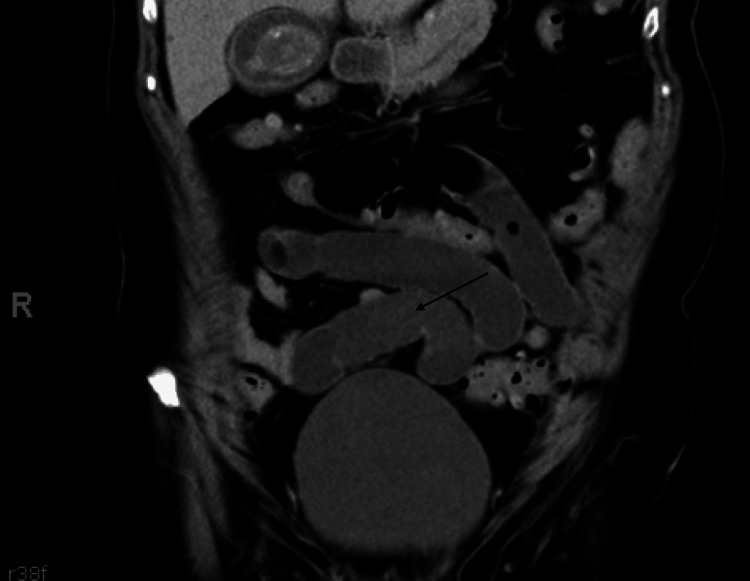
Abdominal and pelvic CT with intravenous contrast from initial presentation (coronal view)
